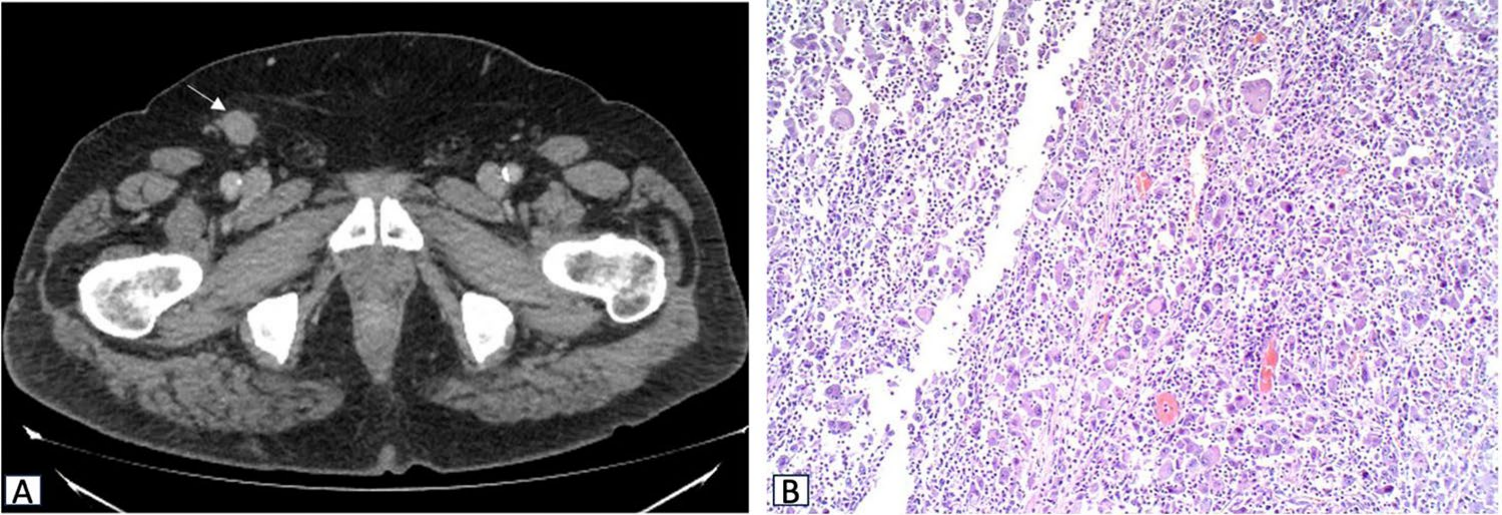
Axial contrast-enhanced CT image in portal phase showing a right inguinal lymphadenopathy (arrow in A), with immunophenotypic features similar to the primary liver lesion (10 × magnification, B)
